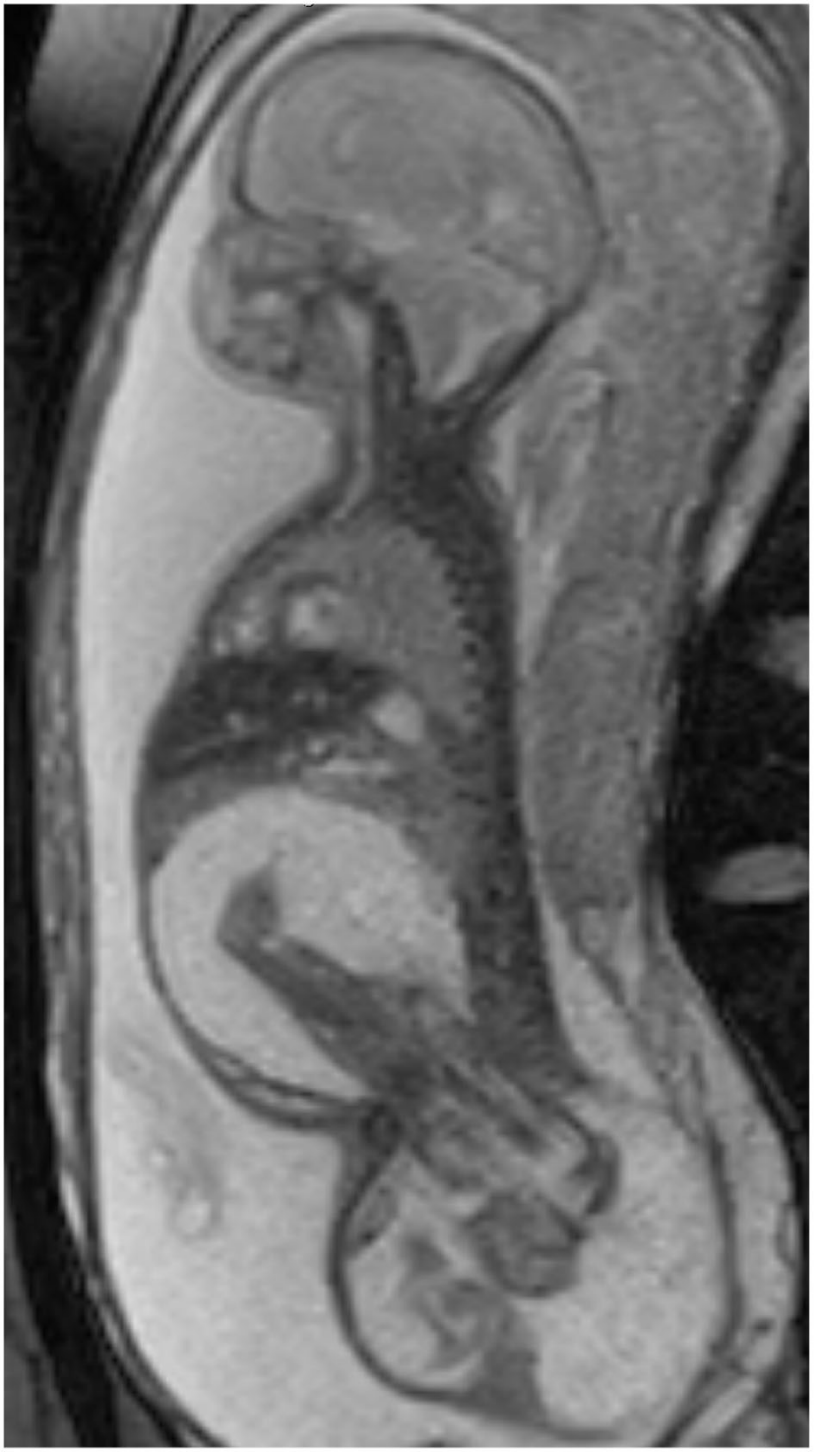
Oblique sagittal T2 weighted fetal MR images demonstrate the large FIF spanning the host twin perineum, with markedly edematous legs of the parasitic twin within the abdominal cavity of the host.
